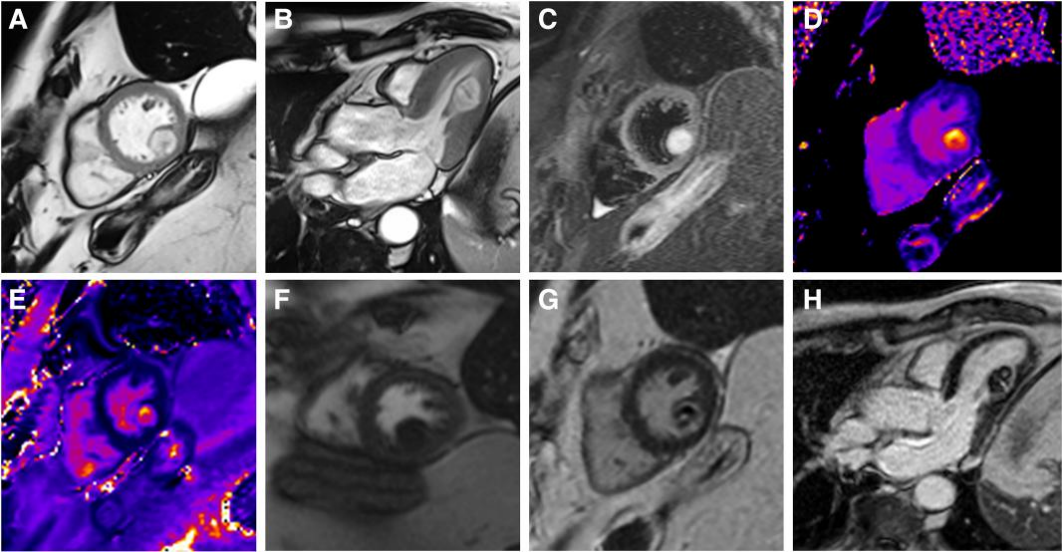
In 2010, a 68-year-old man underwent coccyx resection and radiotherapy because of localized sacral chordoma. In 2015, he received a left superior pulmonary lobectomy because of chordoma metastasis. In 2019, cardiovascular magnetic resonance (CMR) detected a mass at the base of the posteromedial papillary muscle (15 × 14 mm;Supplementary data online, *Figure S1*). In 2023, the patient, still asymptomatic, was referred to us for follow-up CMR because of concerns of mass growth at the latest echocardiography (see Supplementary data online, *Figure S2*, Supplementary data online, *Video S1*). An oval mass contained within the posteromedial papillary muscle with well-defined margins and increased dimensions (26 × 18 mm), as compared with the previous CMR, was seen. In comparison to the myocardium, the mass was hyperintense in cine-SSFP (*Panels A* and *B*; Supplementary data online, *Videos S2* and *S3*), markedly hyperintense in the T2-weighted STIR (*Panel C*) and showed increased T1 and T2 mapping values (*Panels D* and *E*). After contrast injection, there was no increased mass signal at first-pass perfusion (*Panel F*), whereas the late gadolinium sequences revealed soft, heterogeneous enhancement (*Panels G* and *H*). The CMR properties of the mass were unchanged compared with the previous scan and were interpreted as a growing cardiac metastasis of sacral chordoma. Treatment with imatinib was initiated.
